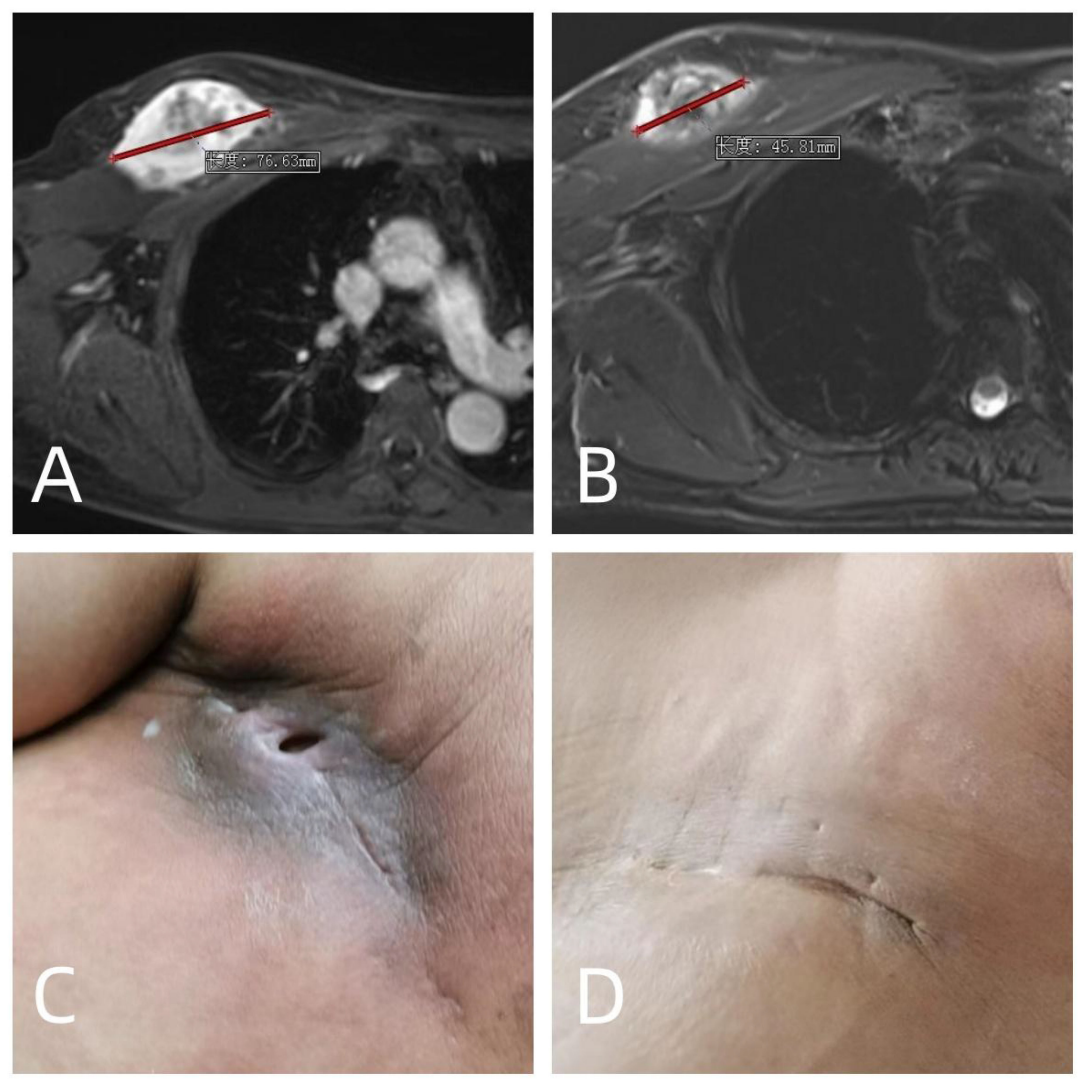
A 58 years old male who diagnosed with soft tissue sarcoma T2N0M0G3 IIIA in the right chest wall was treated with 2 cycles of preoperative doxorubicin and ifosfamide, neoadjuvant radiotherapy 35Gy, and surgery 13 days after neoadjuvant radiotherapy. (A) MRI before neoadjuvant chemoradiotherapy; (B) MRI after neoadjuvant chemoradiotherapy, which were evaluated as PR according to RECIST1.1 criteria; (C) long-term poor wound healing with pain after operation; (D) The patient underwent a second operation 3 months after the first operation. The necrotic area and infected area of the original radiotherapy were cleared, and the pain and infection were cured.
